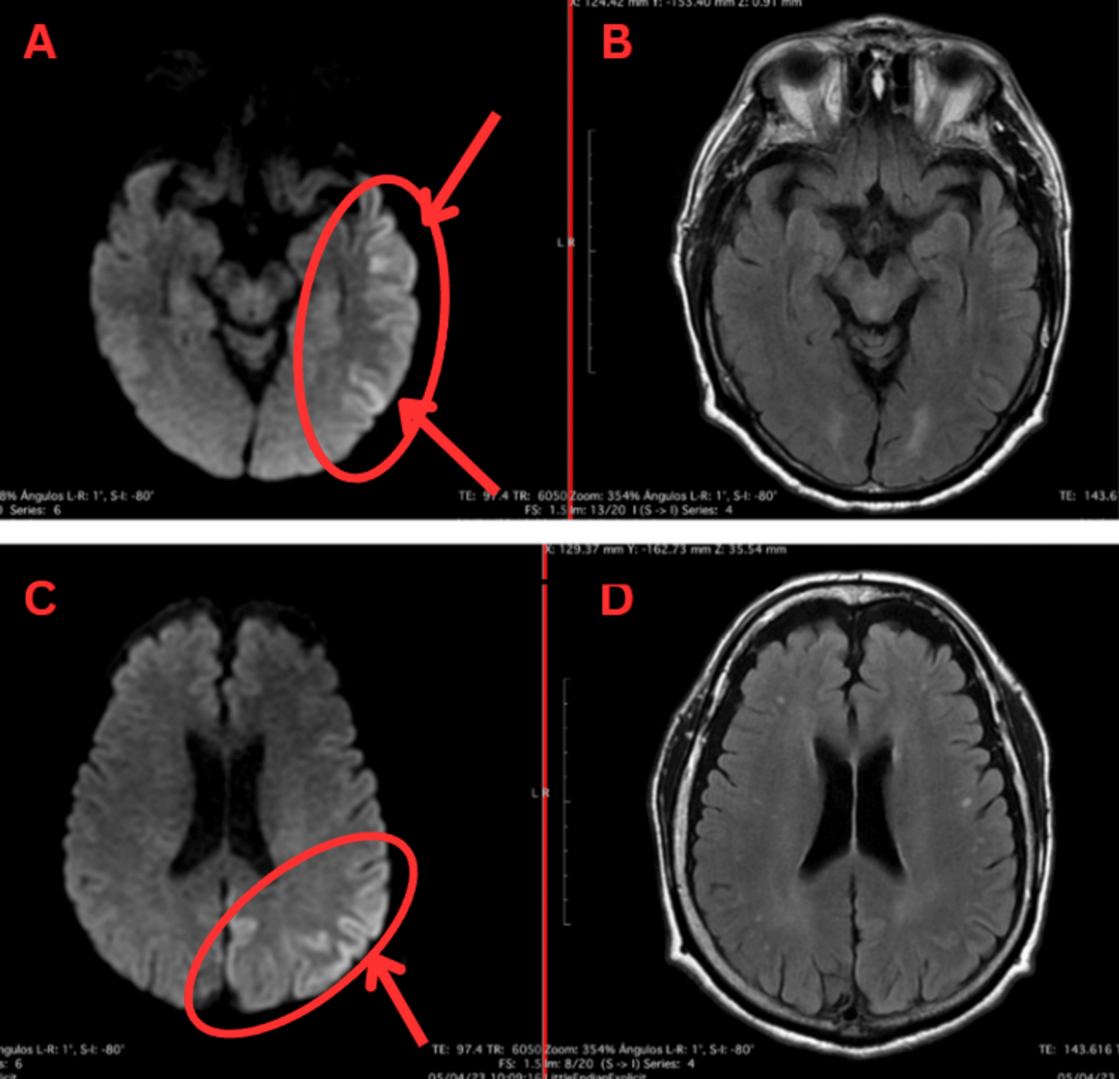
Second axial brain MRI: diffusion-weighted and fluid-attenuated inversion recovery (FLAIR) sequences Panels A and C show persistence of inflammatory changes in the left temporal and parietal cortex compared to the previous study. Panels B and D (FLAIR sequences) do not yet show significant changes.
